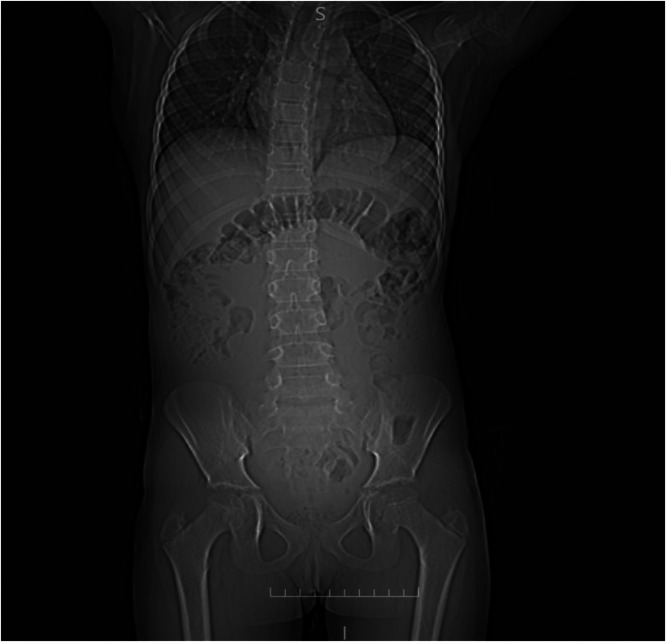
Supine abdominal radiograph demonstrating a large soft-tissue opacity with upward displacement of gas-filled transverse colon and nondilated small-bowel loops.
